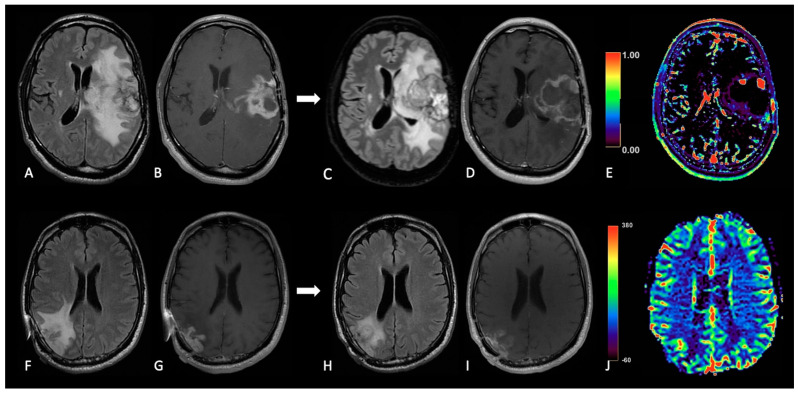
TP and pseudoresponse after BEV. Upper row: MRI at baseline and after 6 months of BEV. FLAIR (A,C), post-contrast T1w images (B,D), and DCE-Ktrans map (E). At follow-up there are increase in FLAIR signal intensity and enhancing tumor components, with high values of Ktrans in the solid components, suggestive of TP. Lower row: MRI at baseline and after 5 months of BEV. FLAIR (F,H), post-contrast T1w images (G,I) and DSC-CBV perfusion map (J). Follow-up MRI shows the reduction in FLAIR signal intensity and CE, with high rCBV values, characteristics of pseudoresponse.
